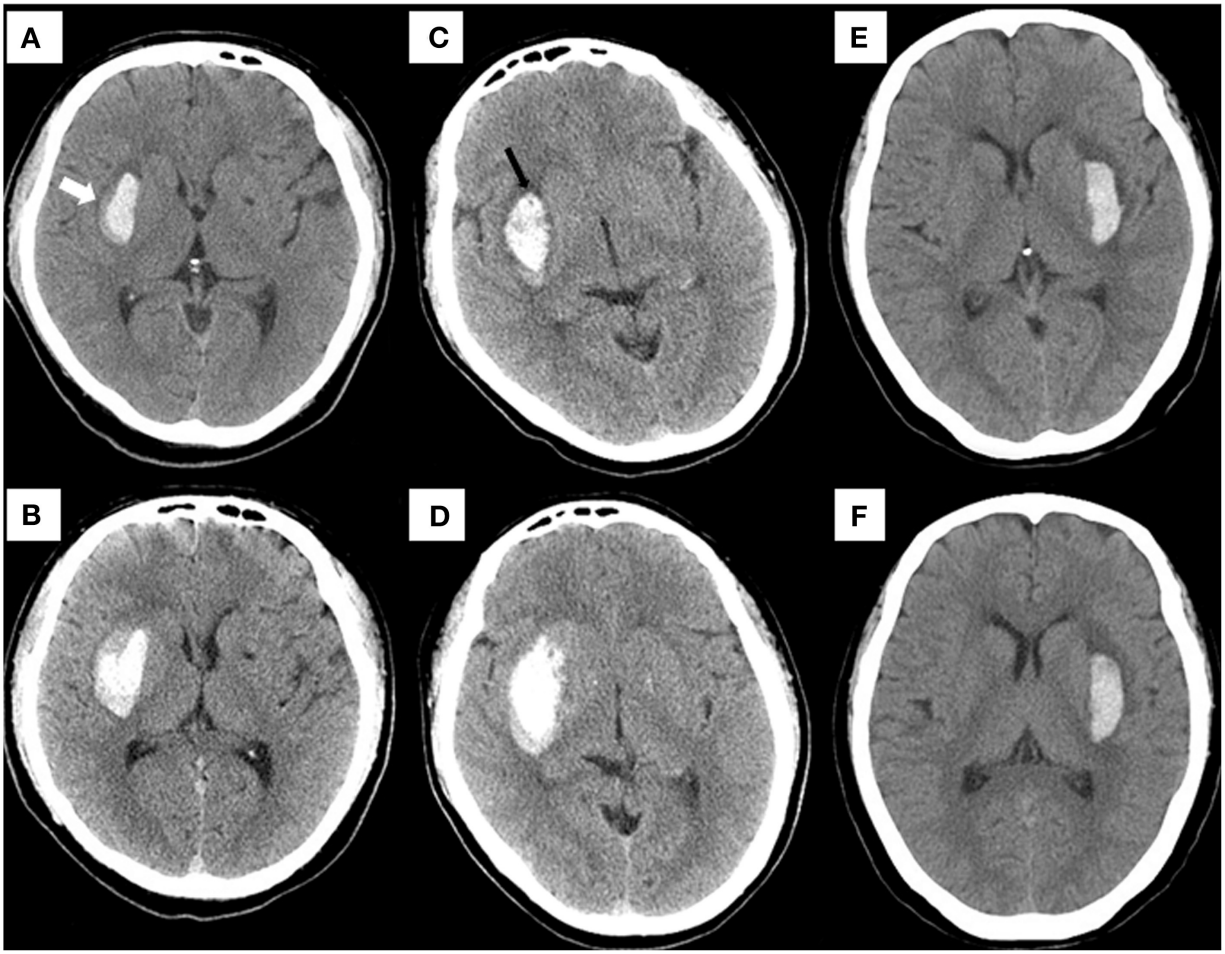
Examples of using the nomogram to predict the risk of HE. Case 1: (A) a patient who underwent cranial NCCT within 1.5 h of onset presented with a hematoma (6.1 ml) in the basal ganglia. The risk of HE estimated by nomogram was approximately 71.36% (blend sign = present, hypodensities = absent, NLR = 10.22, time to initial NCCT <3 h, R-score = −0.21, total points = 134, and estimated HE risk = 71.36%). A blend sign (white arrow) can be seen. (B) The hematoma volume was enlarged to about 11.8 ml on the follow-up CT at 8 hours. Case 2: (C) a patient who underwent cranial NCCT within 2.5 h of onset presented with a hematoma (10.1 ml) in the basal ganglia. The risk of HE estimated by nomogram was approximately 70.78% (blend sign = absent, hypodensities = present, NLR = 13.03, time to initial NCCT <3 h, R-score = −0.19, total points = 131, and estimated HE risk = 70.78%). A hypodensity sign (black arrow) can be seen. (D) The hematoma volume was enlarged to about 24.8 ml on the follow-up CT at 10 Hours. Case 3: (E) using this nomogram, the hematoma (4.8 ml) on the initial NCCT showed a low expansion risk (blend sign = absent, hypodensities = absent, NLR = 2.52, time to initial NCCT>3 h, R-score = −1.59, total points = 30, and estimated HE risk = 1.99%). (F) At 22.5 h after onset, the follow-up CT detected a hematoma of similar size (5.2 ml). NCCT, noncontrast computed tomography. HE, hematoma expansion.
